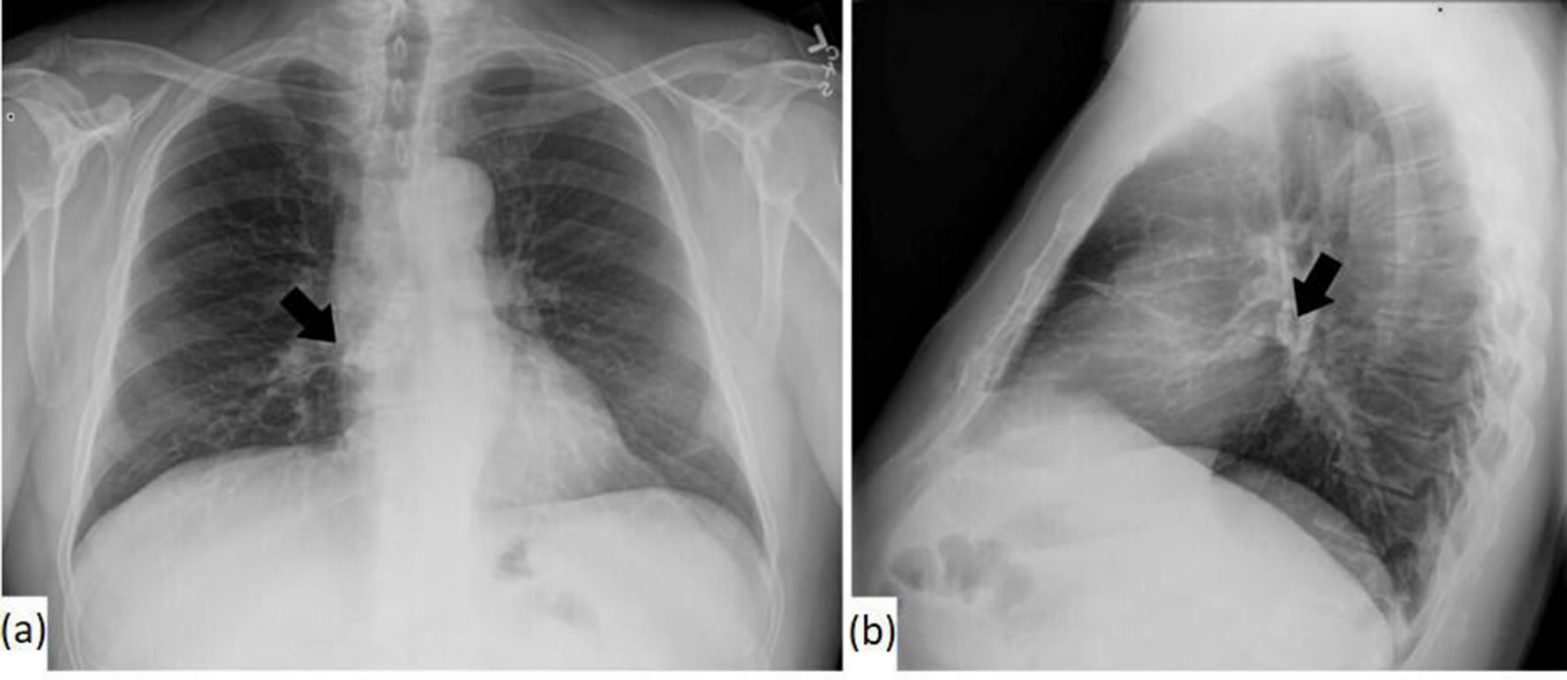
Chest x-ray: (a) posteroanterior and (b) lateral. Small calcified nodes were present in the inferior right hilum and subcarinal chain (indicated by the arrow). No suspicious pulmonary nodules nor infiltrates were identified.
